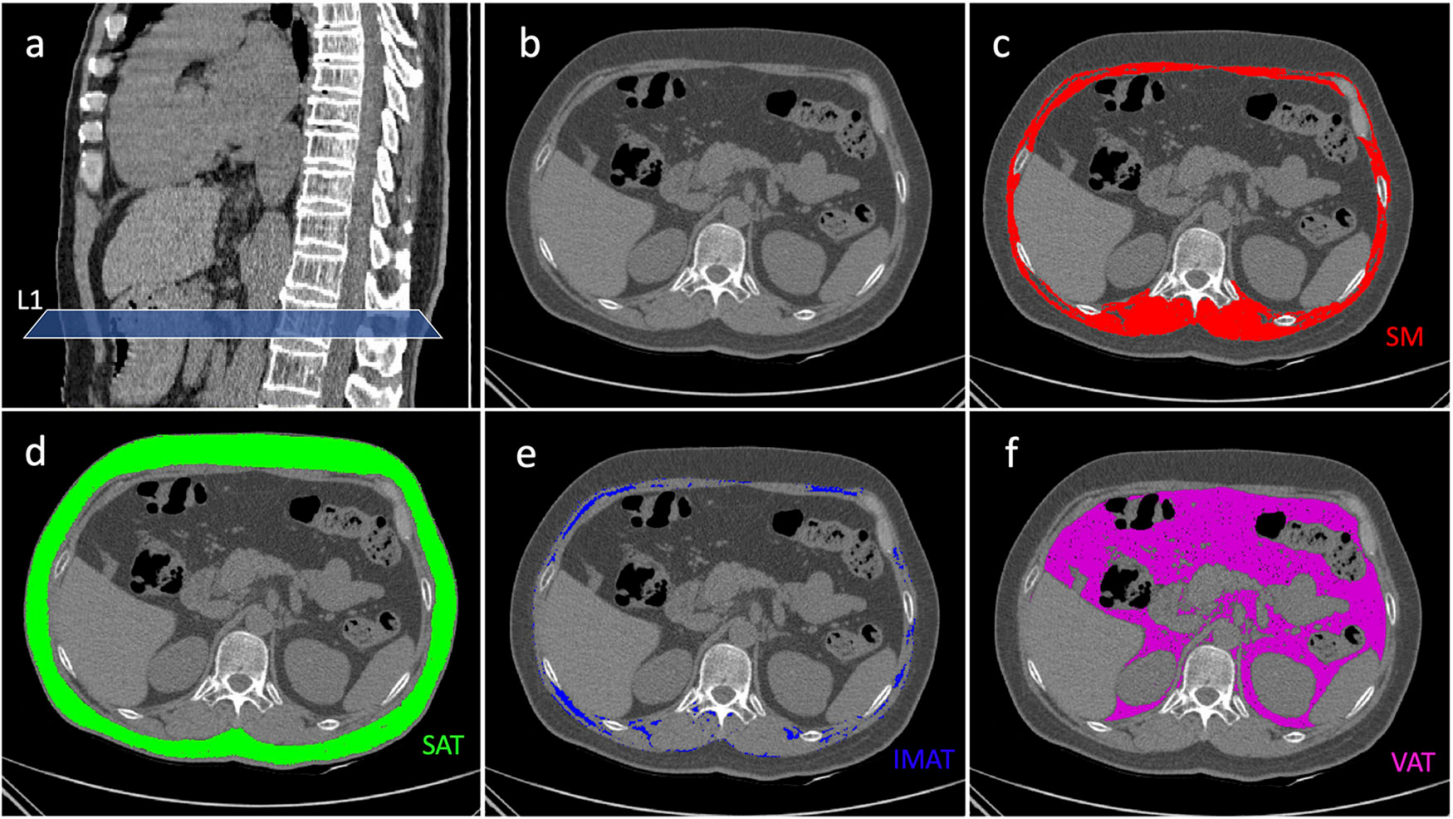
Segmentation of body composition at the level of L1 based on CT image. a Body composition segmentation at L1 level. b Unsegmented CT image. c Segmented skeletal muscle (SM, red). d Segmented subcutaneous fat (SAT, green). e Segmented intermuscular adipose tissue (IMAT, blue). f Segmented visceral adipose tissue (VAT, purple)
